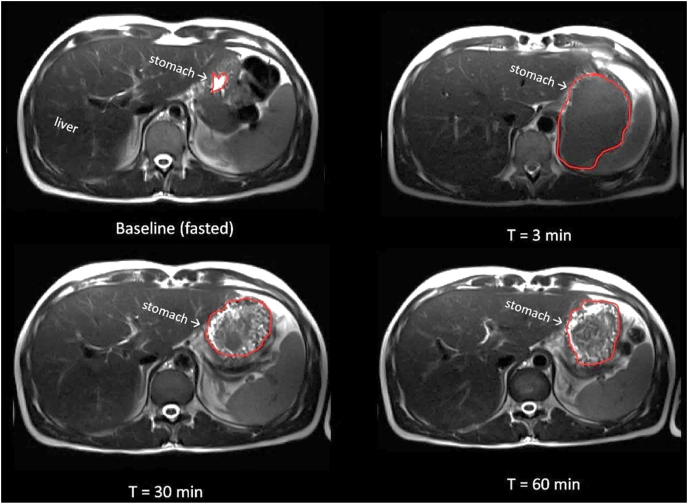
Examples of T_2_-weighted MRI images showing cross-sections through an empty stomach after an overnight fast (baseline) and after 300 mL casein drink consumption. At *t* = 30 and 60 min, coagulation can be observed. MRI, magnetic resonance imaging.
